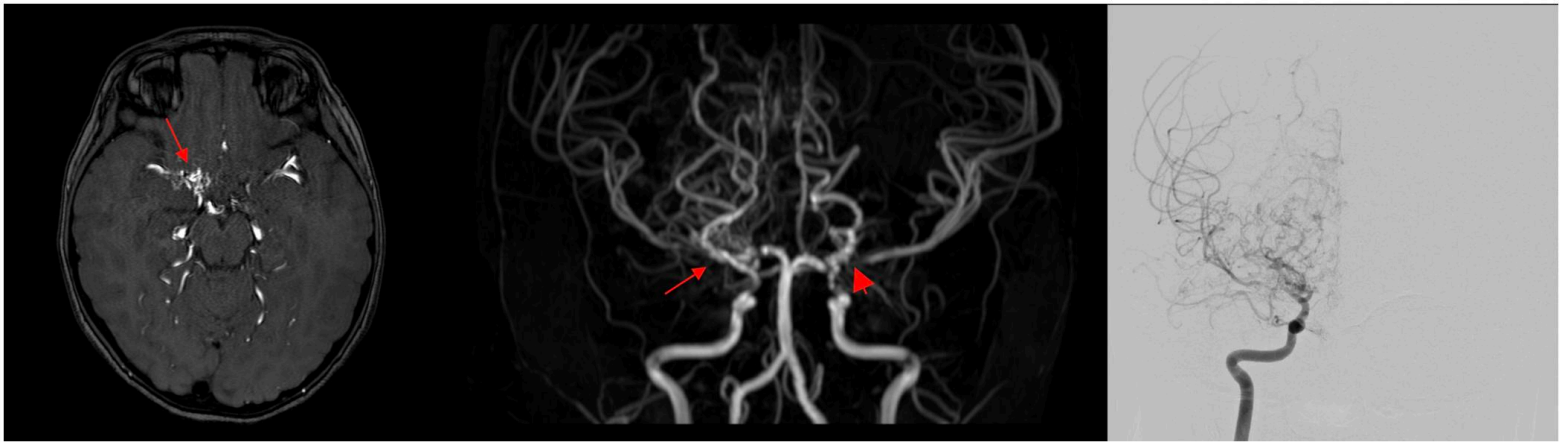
T1-weighted and 3D time-of-flight (TOF) MR angiogram—severe stenosis in M1 segment of right middle cerebral artery, and puff-of-smoke appearance in right lenticulostriate arteries (red arrow). Left M1 segment stenosis is less involved (arrowhead). Digital subtraction angiogram of right internal carotid artery shows severely attenuated M1 segment with opening of collaterals in right lenticulostriate perforators, compatible with Moyamoya phenomenon.
